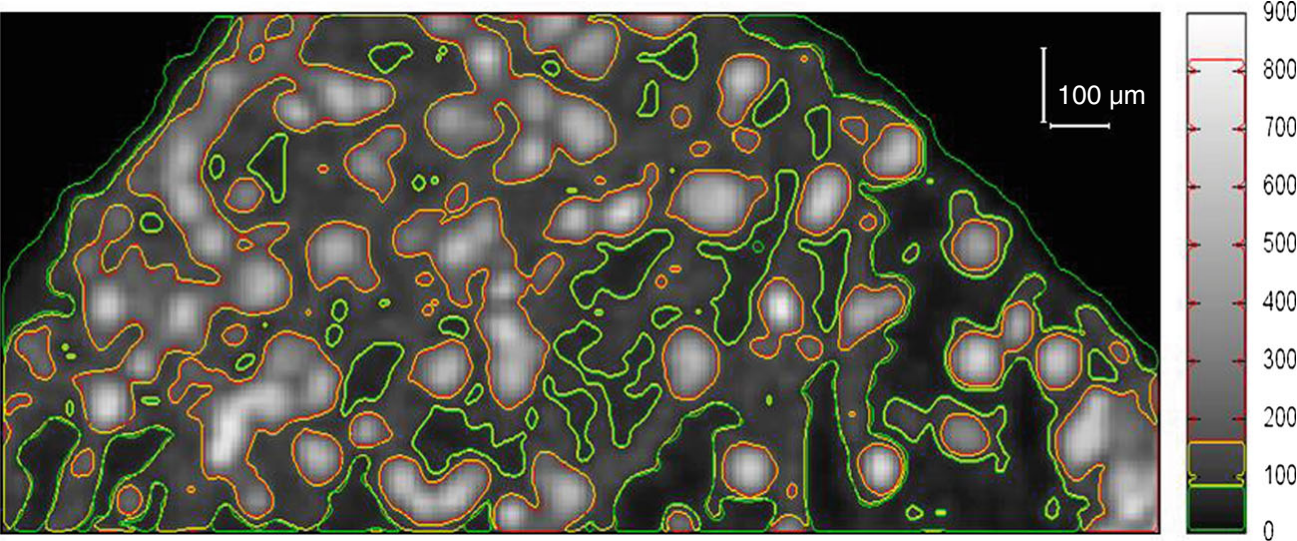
An example of the XRF map of chromium distribution in the *C. cophocarpa* mature leaf incubated in Cr(III) used for analysis on Image-Pro Plus software. *Different colors* point out areas of different Cr accumulation. The *black* and *white* scale on the right indicates X-ray intensity for Cr in counts per second (cps)
